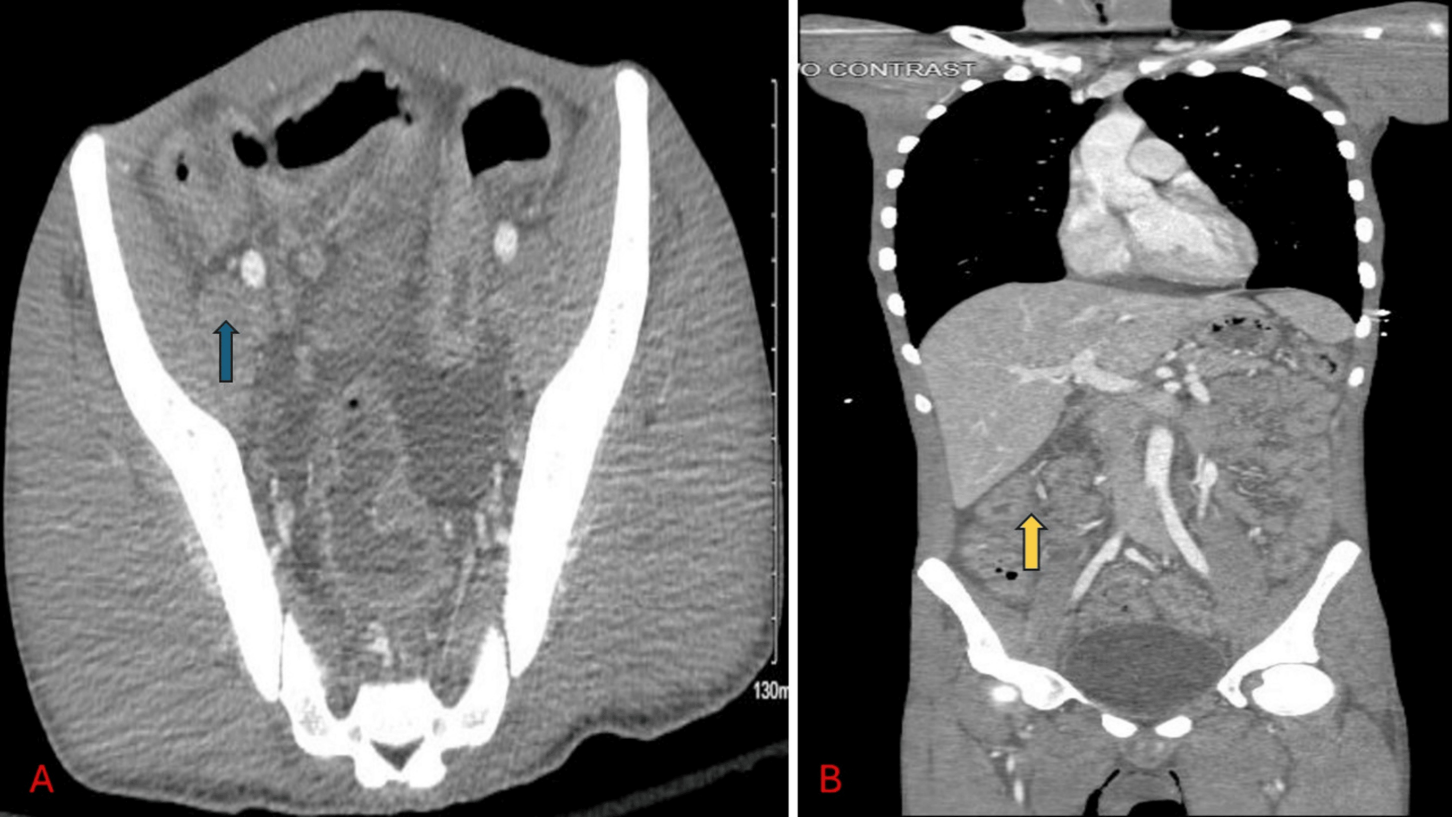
Computed tomography (CT) scan of abdomen and pelvis of the patient A) Blue arrow in A shows colon wall enhancement with haziness and thickening which is consistent with possible inflammation, most likely colitis; B) Yellow arrow in B shows ascending colon wall enhancement and thickening suggestive of colitis
